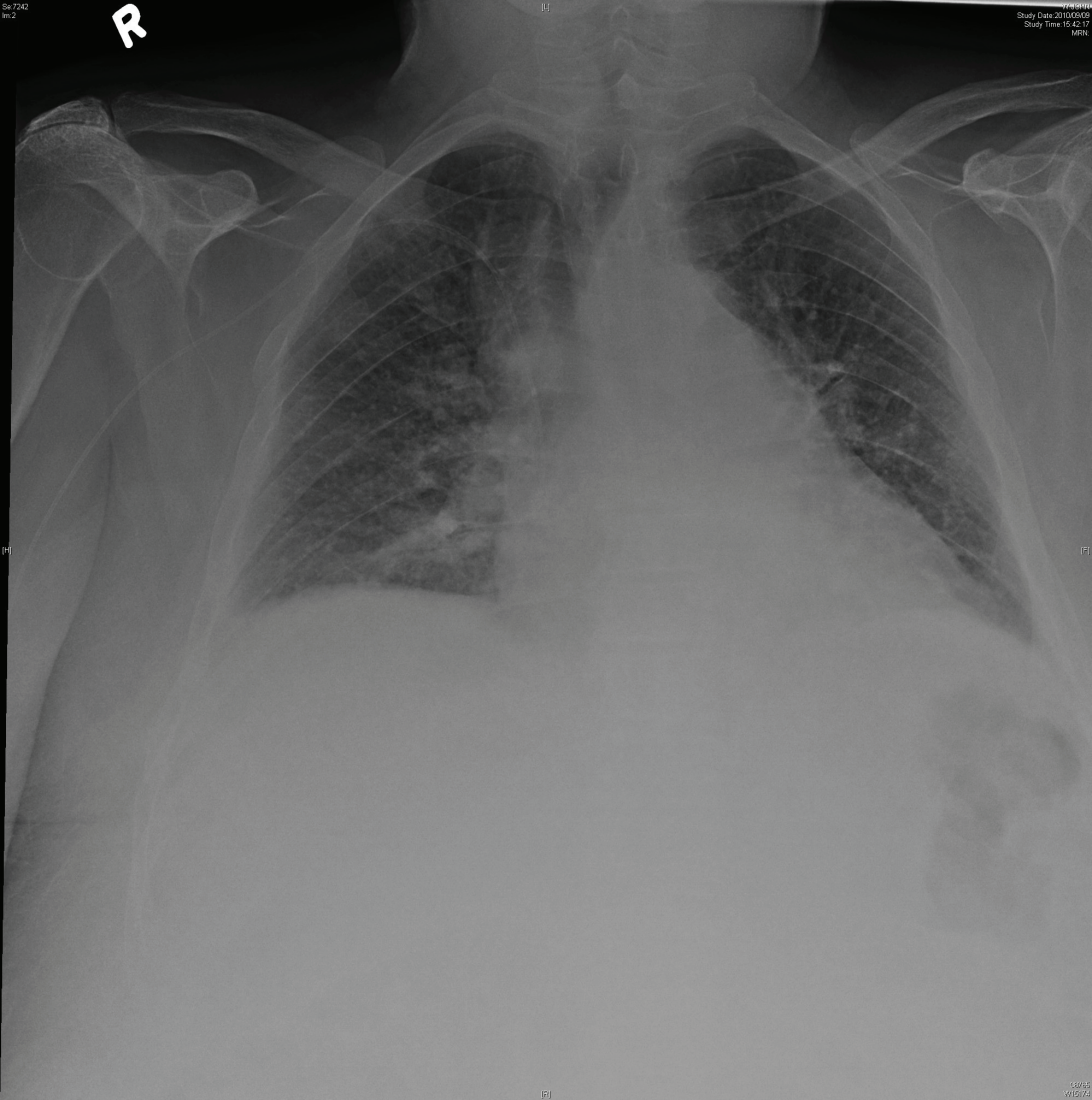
Chest X-ray showing an interstitial pattern suggestive of eosinophilic pneumonitis in this patient.
